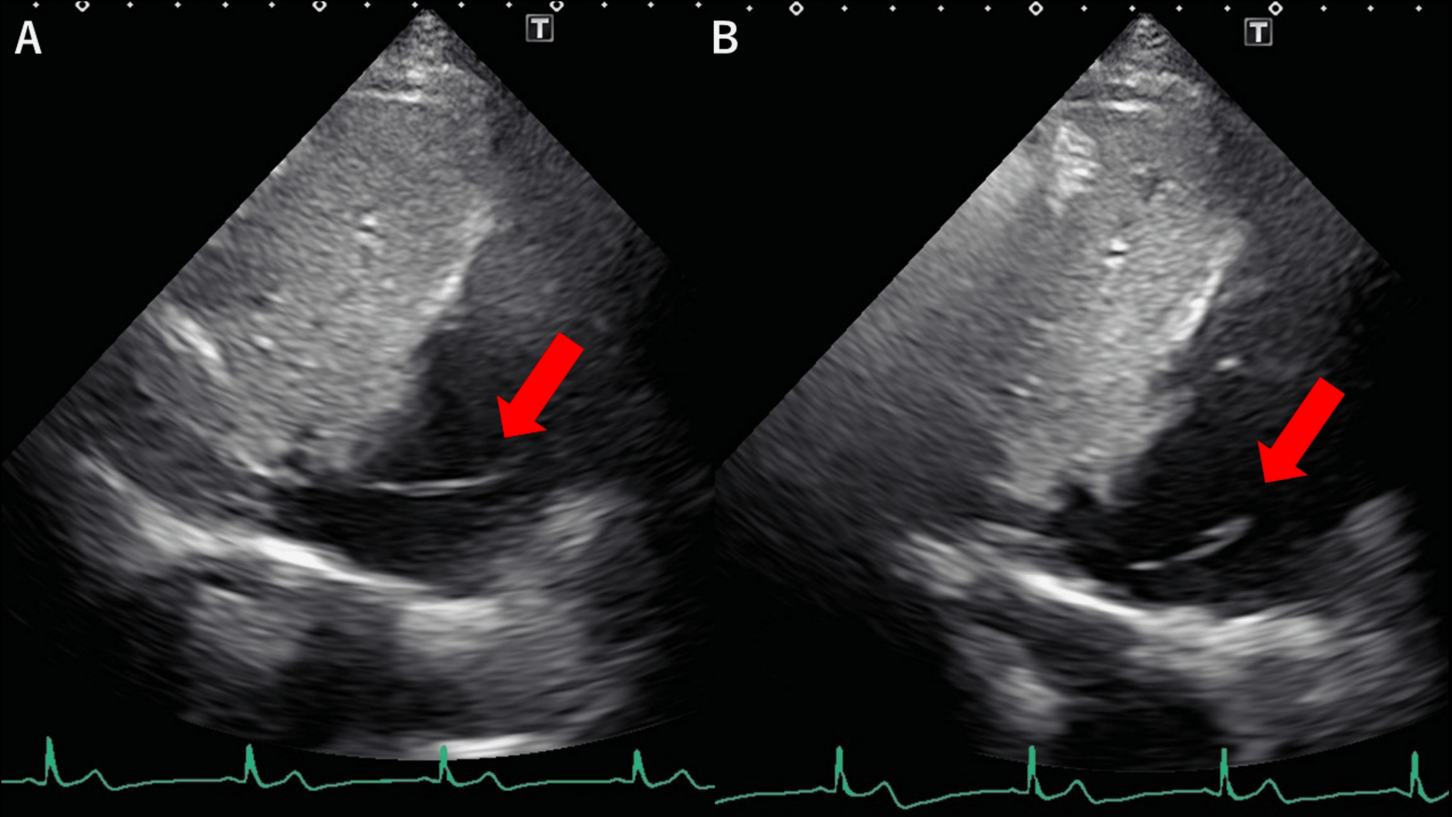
Eustachian valve in the right atrium Transthoracic echocardiography showing IVC. A string-shaped hyperechoic structure (red arrow) is shown in the right atrium near the opening of the IVC. This structure moves up (A) and down (B). It is less mobile than the ribbon-shape structure in Figure 1.
